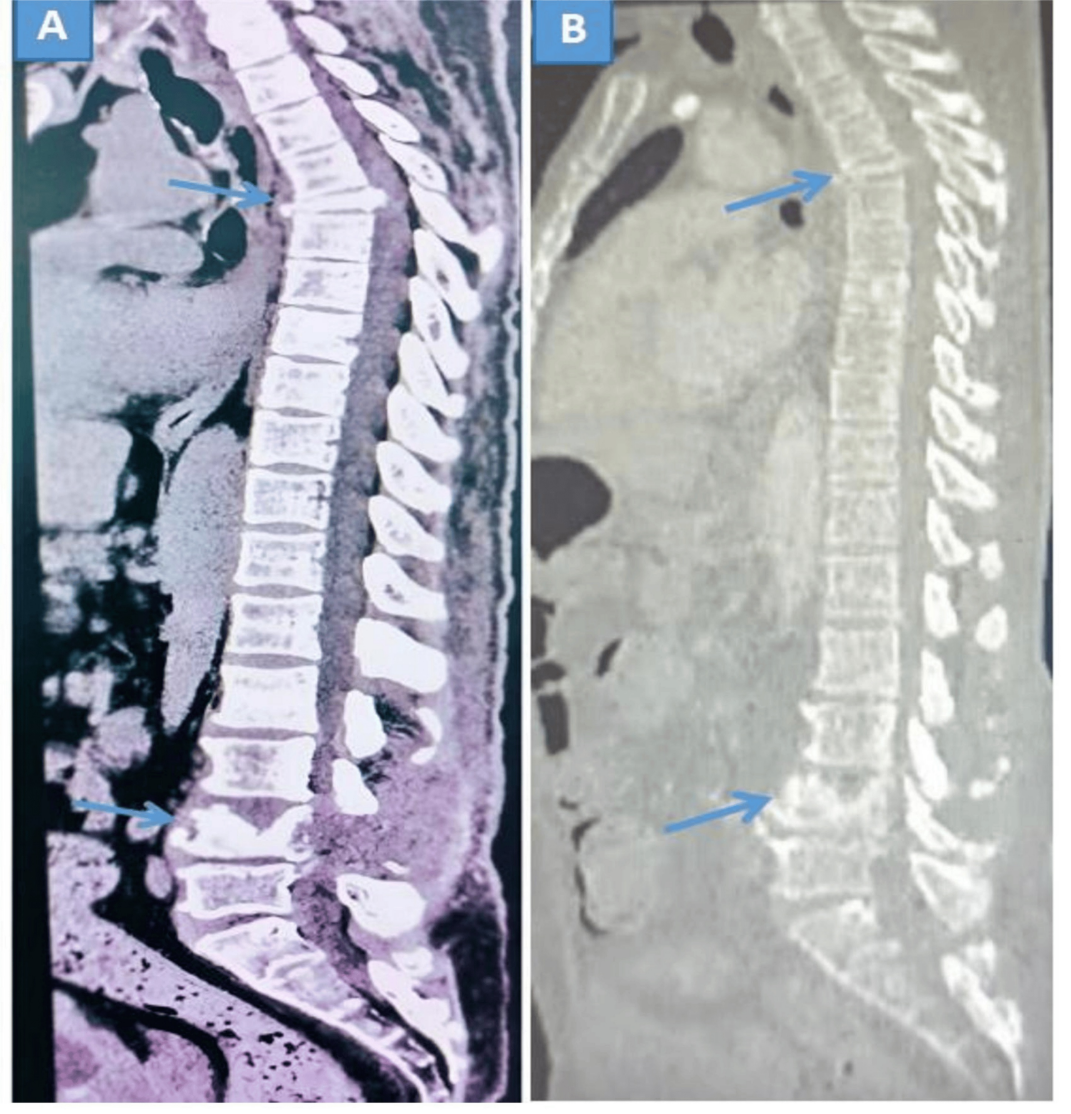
(A) Soft tissue and (B) bone windows of the spinal sagittal section CT scan image showing the thoracic (T5) and lumbar (L4) localization of prostate carcinoma metastases.
